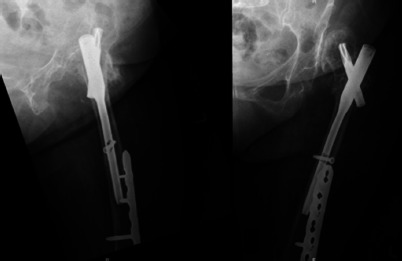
Post-operative radiographs showed the left hip fracture has well healed. No evidence of implant loosening.
